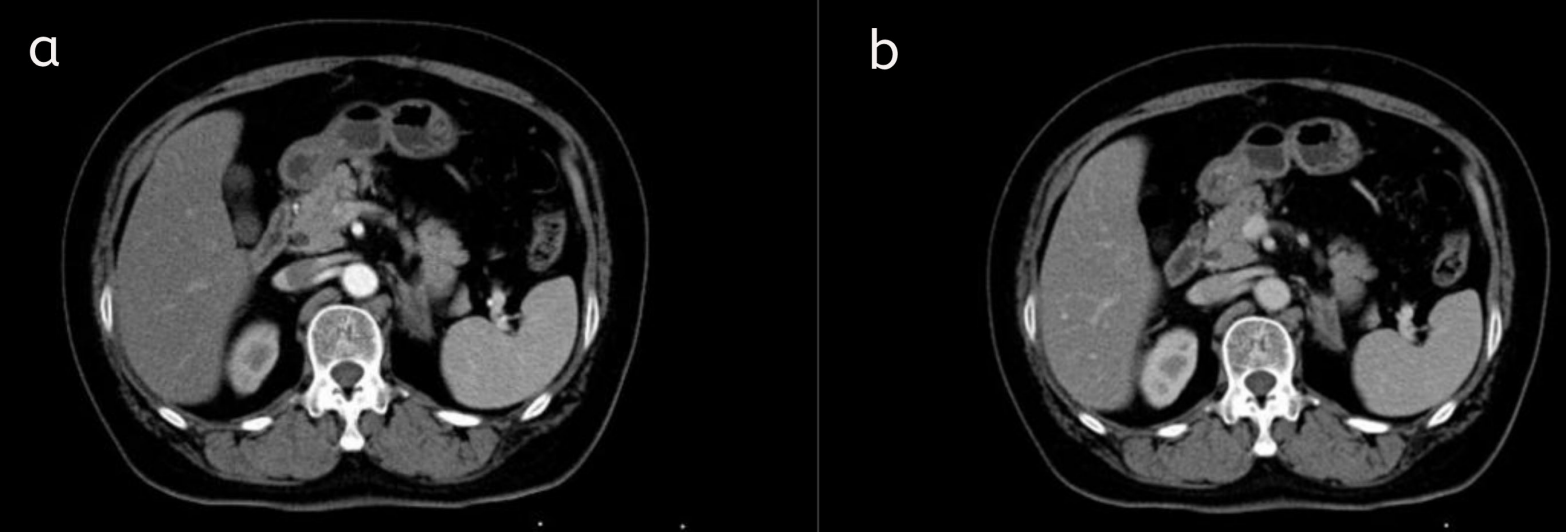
Post-treatment follow-up CT images: arterial phase (a) and venous phase (b). Following standard lymphoma therapy with the R-CHOP regimen, follow-up abdominal CT demonstrated a reduction in the size of the left adrenal lymphoma lesion (decreased to approximately 3.4 cm × 1.8 cm) compared to pre-treatment imaging. The mass effect of the original lesion was alleviated, and its degree of enhancement was reduced. These imaging findings are consistent with a positive treatment response.
